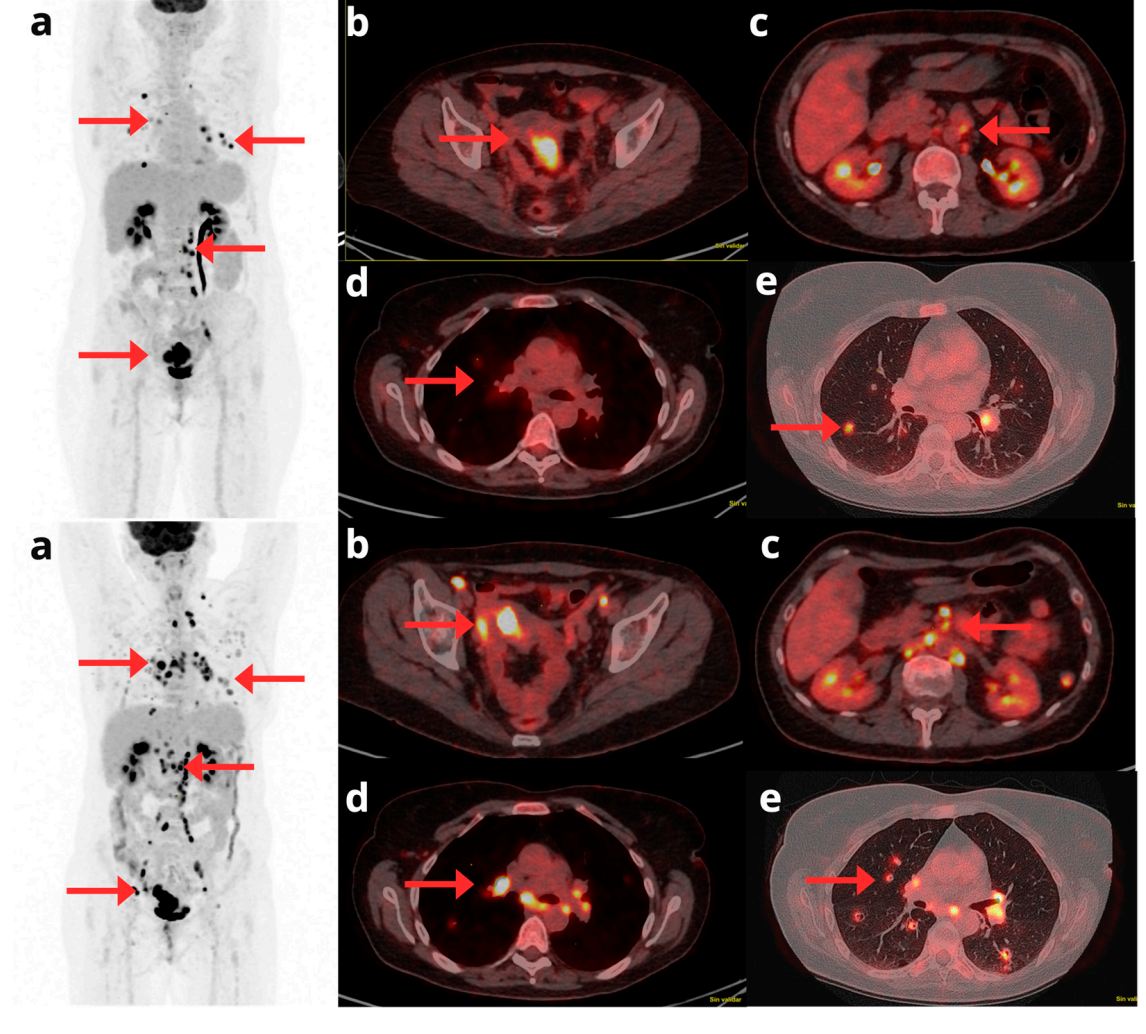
A 58-year-old woman diagnosed with stage IV clear cell EC, undergoing treatment with Pembrolizumab and Lenvatinib. A comparison is presented between the pre-treatment [^18^F]F-FDG PET/CT (top row) and follow-up study (bottom row). The maximum intensity projection PET images (a) provide a global view confirming clear disease progression. This progression is evident through both an increase in size and metabolism of known lesions and the appearance of multiple new metastatic foci (red arrows). Axial slices detail these findings, showing increased pelvic involvement (b), enlarged supra and infradiaphragmatic lymphadenopathies (c,d), and the appearance of new bilateral pulmonary nodules (e).
